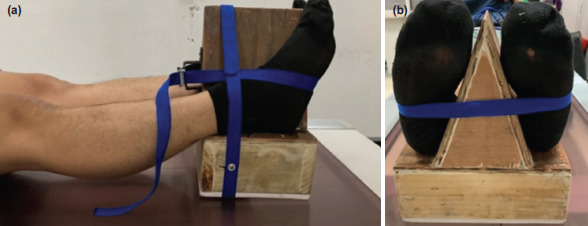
Radiograph positioning by Hip internal rotation assistive device. (a) Sagittal view and (b) axial view.
